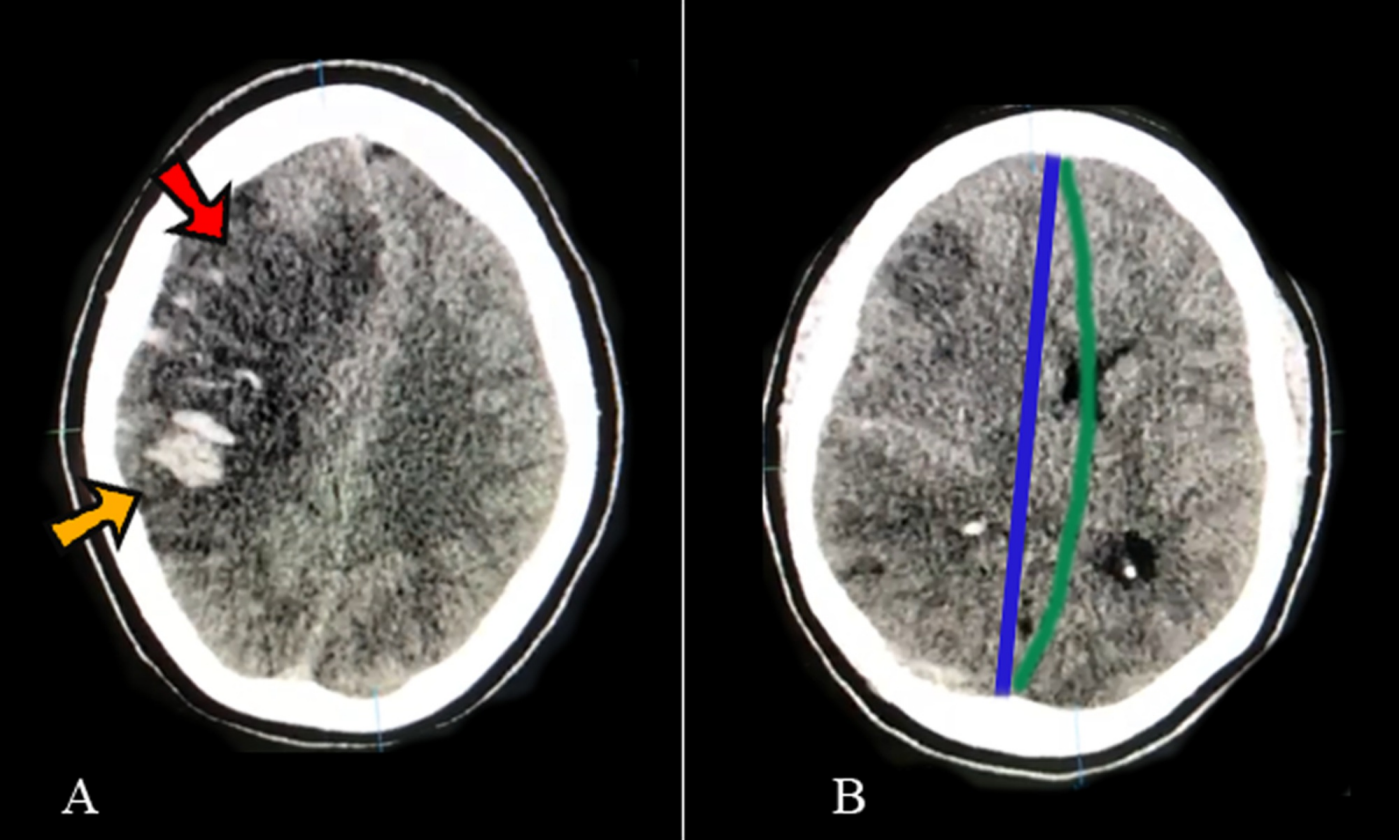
Pre-operative computerized tomography (CT) scans Pre-operative computerized tomography scans show on A: Large frontoparietal hypodensity (red arrow) with hyperdense areas (yellow arrow) suggestive of ischemic stroke with hemorrhagic transformation and on B: Midline shift measuring 8.5mm, suggestive of cerebral edema (>5mm). The midline is marked with a blue line and the midline shift with a green line. All of these can be indirect signs of cerebral venous thrombosis (CVT)
